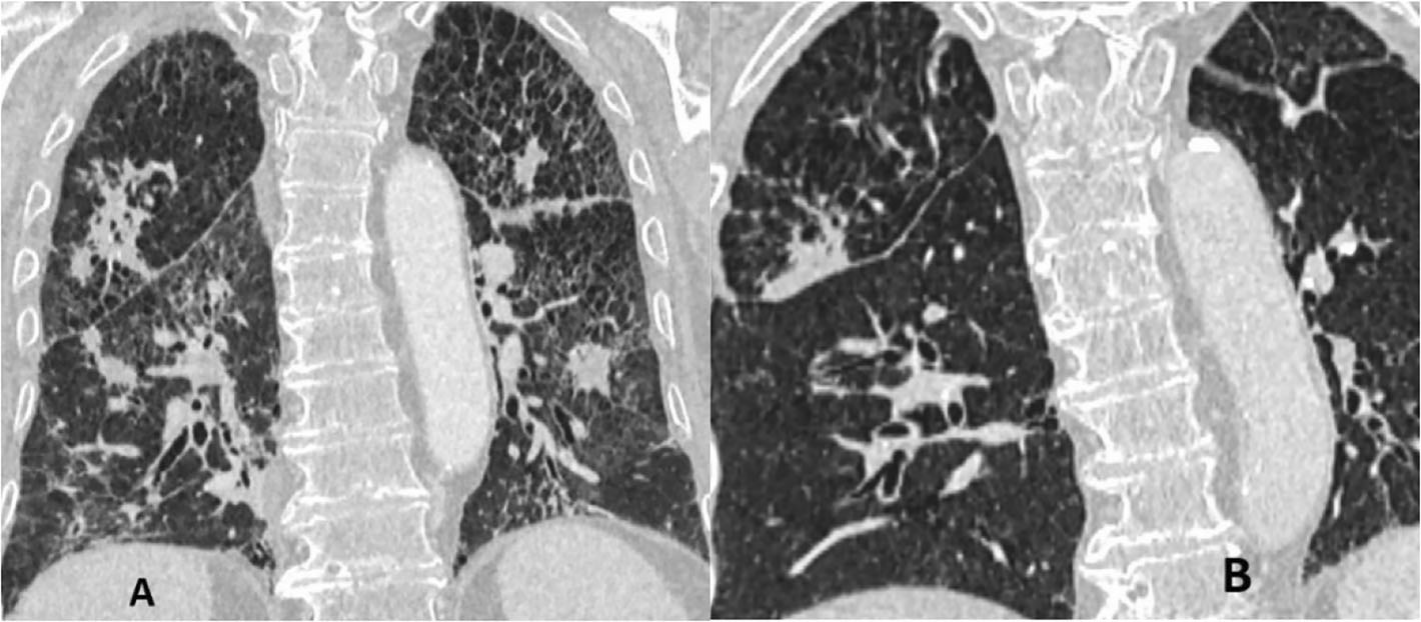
Coronal reconstruction of chest HRCT before (a) and after (b) the treatment
